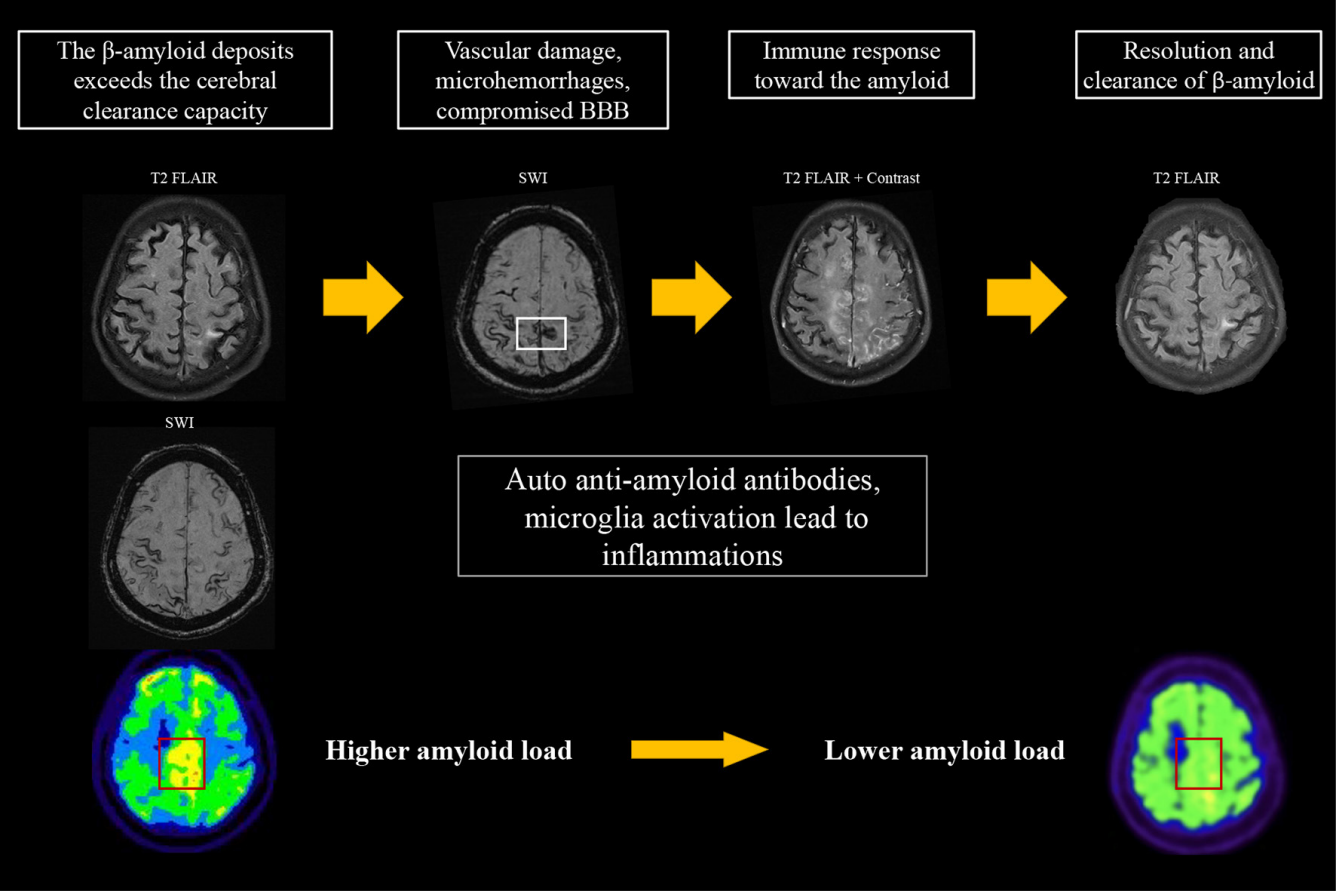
Proposed hypothesis for the changes on amyloid PET in CAA-ri. An increase in amyloid burden exceeds the clearance capacity, which may lead to focal vascular damage and microhemorrhages. This process induces an immune response that involves auto-antibodies and activation of microglia/macrophages for the clearance of β*-*amyloid, resulting in the typical MRI features of CAA-ri. The focal amyloid load appears lower when inflammation resolves.
